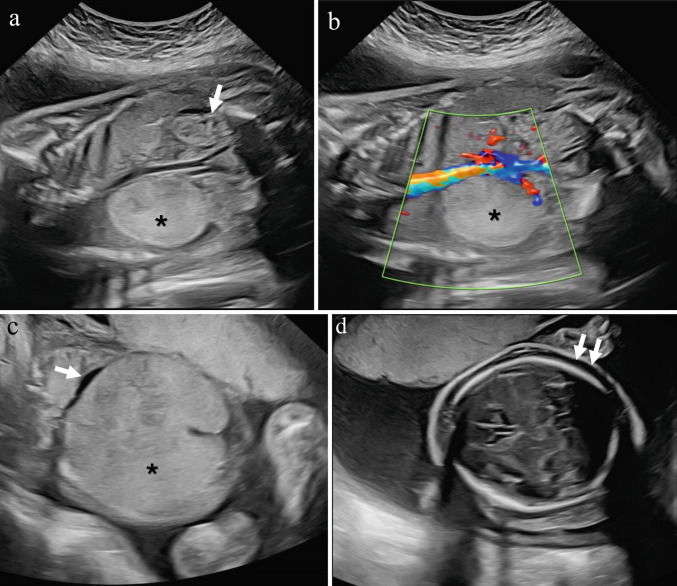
Ultrasound images of a 26-week gestational age male fetus with congenital neuroblastoma and placental metastases. a Grayscale longitudinal ultrasound image of the fetal abdomen reveals a well-defined, oval-shaped homogeneous hyperechoic lesion (*asterisk*) in the right suprarenal region, inseparable from the right kidney. The left kidney appears normal (*arrow*). b Longitudinal doppler image of the lesion (*asterisk*) shows no appreciable internal vascularity. c Grayscale longitudinal image of the fetal liver demonstrates hepatomegaly with mild heterogeneous echotexture and no focal lesions (*asterisk*), and mild ascites (*arrow*). d Grayscale transverse image of the fetal brain demonstrates diffuse scalp edema (*arrows*)
